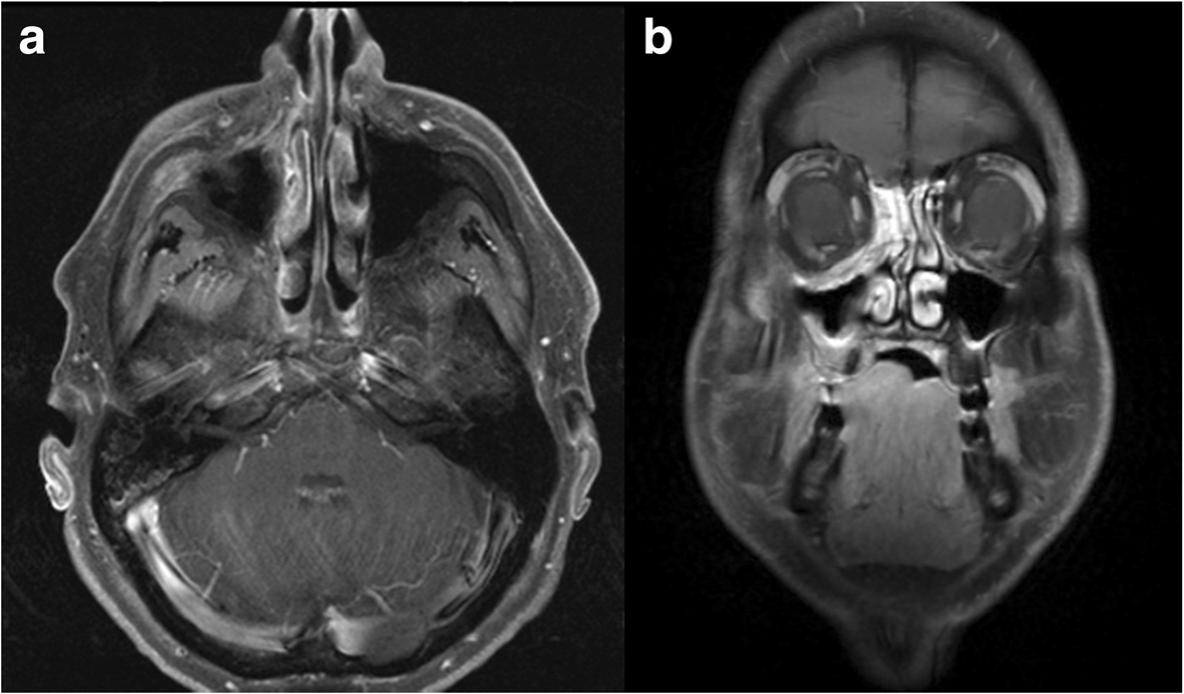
Follow-up contrast-enhanced T1-weighted and fat-saturated MRI scan of the patient with mucoepidermoid carcinoma of the paranasal sinus 6 weeks post completion of radiotherapy (a: axial; b: coronal)
